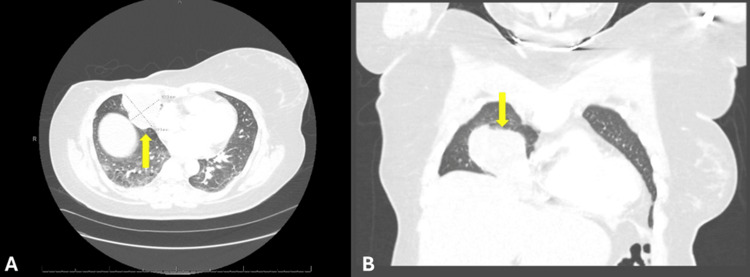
Cross-sectional computed tomography angiography (CTA) imaging of the chest and abdomen with IV contrast showing lung metastasis. (A) An axial view and (B) a coronal view displaying a 6.3×5.2 cm lesion (yellow arrow) abutting the anterior right chest wall.
